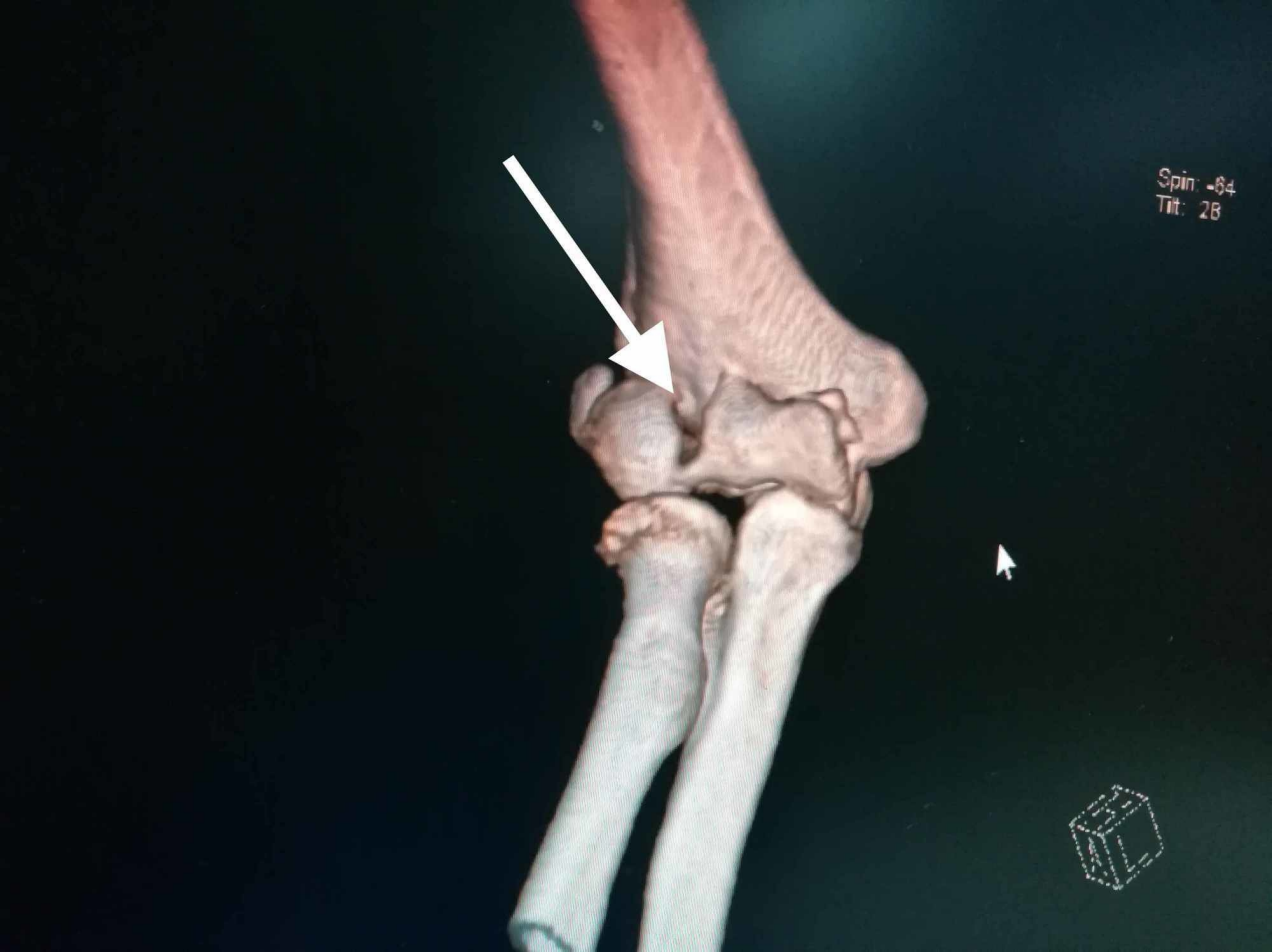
Computed tomography (CT) scans with a 3D reconstruction helps in the better assessment of the fracture profile
